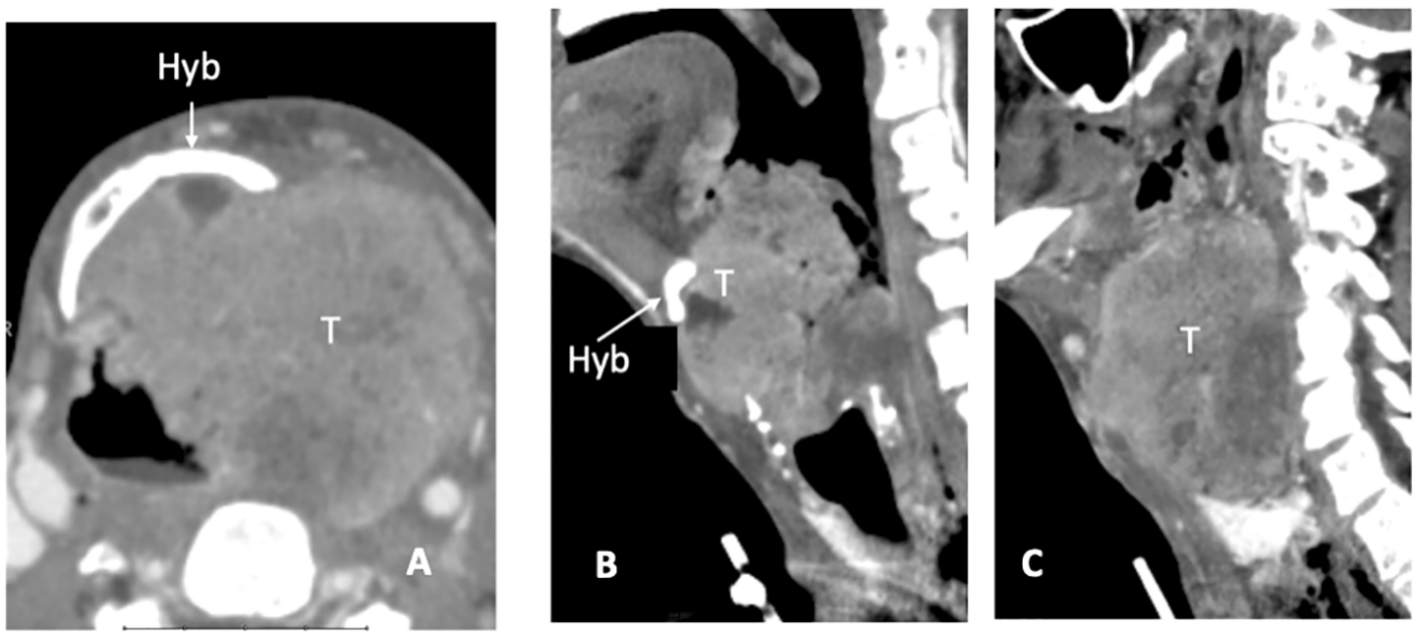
CT scan, axial plane (A), at the supraglottic level, a bulky laryngeal cancer (T) invades the left side of the hyoid bone (Hyb) and presents massive extra-laryngeal spread. On sagittal plane (B) the median part of the hyoid bone is detectable (white arrow), while in the second (left paramedian) image the left portion is replaced by tumour tissue (C).
